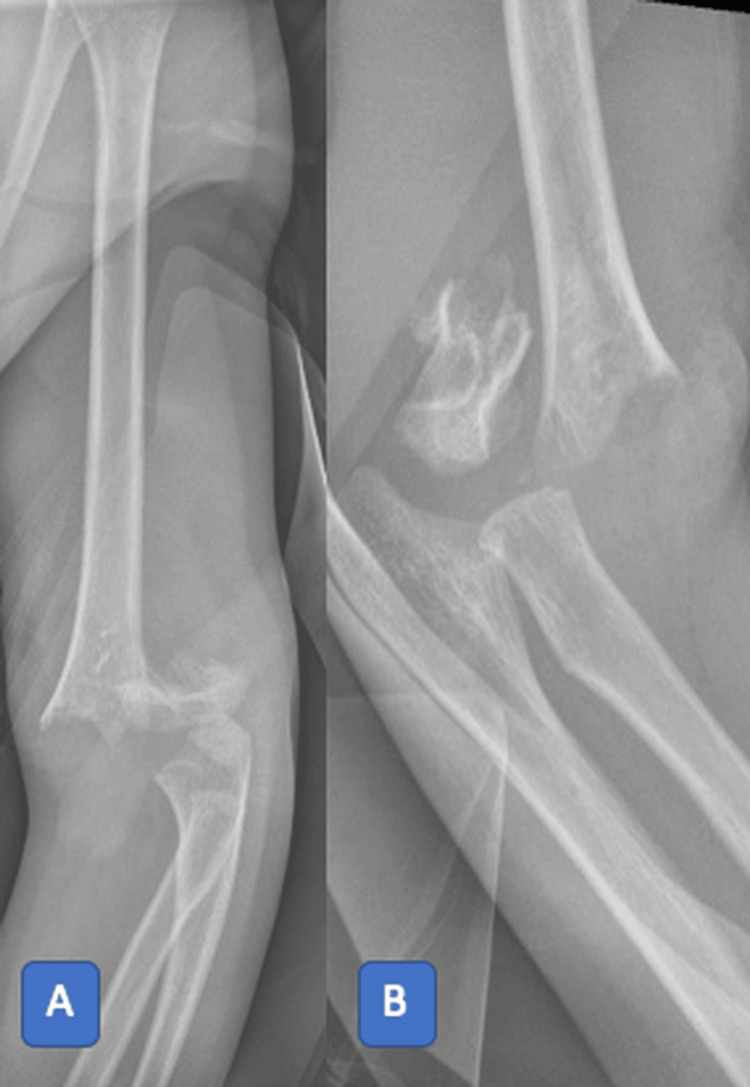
Anteroposterior (A) and lateral (B) radiographs demonstrating a displaced Gartland type III supracondylar fracture of the left humerus with posterior displacement of the distal fragment.
